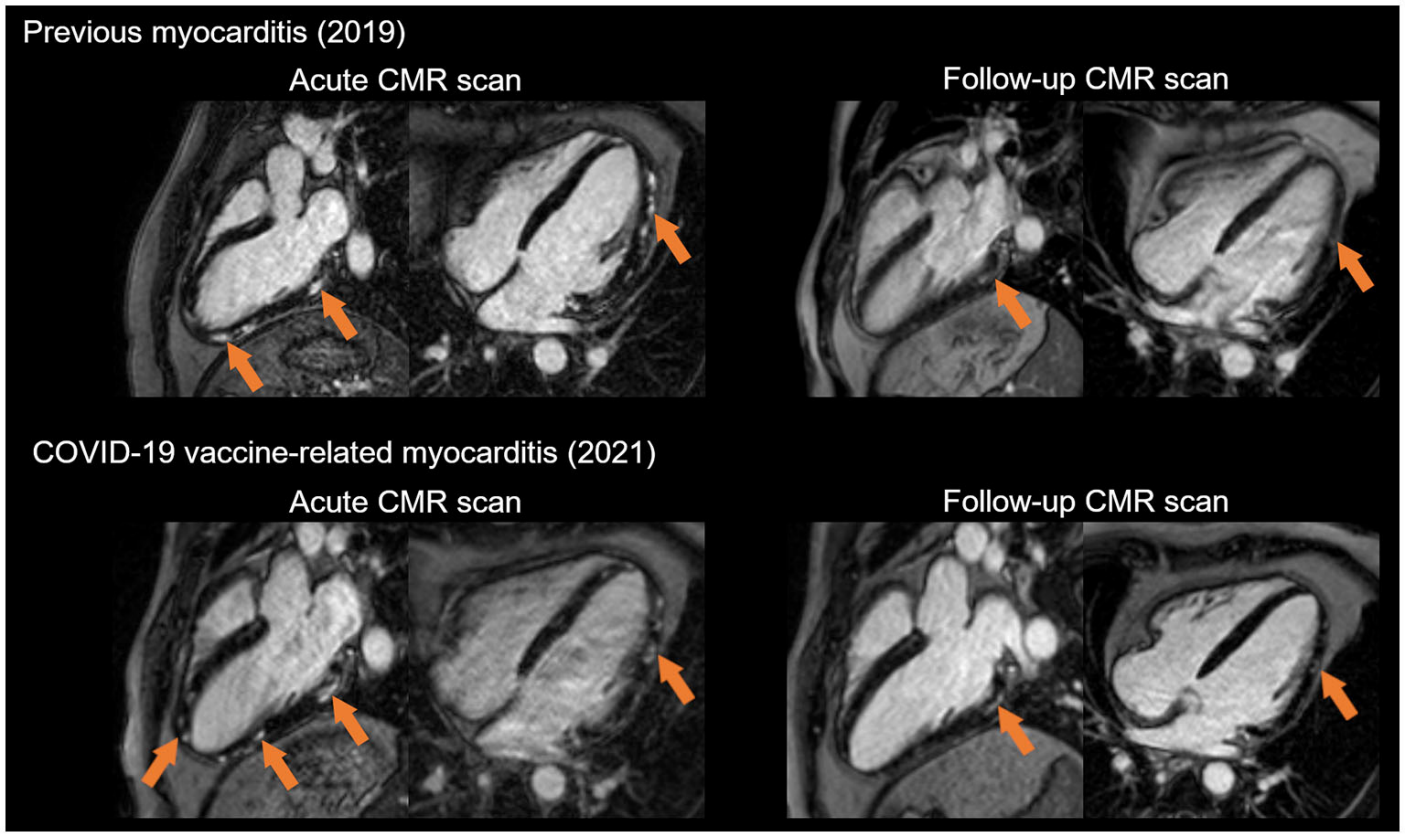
Recurrent myocarditis in a young male patient after the second dose of anti-COVID-19 vector vaccine. Our patient had prior myocarditis in 2019. At the time, he presented with chest pain preceded by gastrointestinal infection and fever. He had elevated troponin levels, and the CT coronary angiogram was negative. The acute CMR showed patchy subepicardial oedema and late gadolinium enhancement (LGE) (orange arrows). Three months later, on his follow-up scan, the oedema disappeared, and the LGE shrank. In 2021, the patient experienced fever and recurrent chest pain 2 days after the second dose of the COVID-19 vaccine. His acute CMR imaging showed LGE in a similar pattern as during the first acute myocarditis episode. Notably, signs of myocardial injury resolved on the follow-up scan.
